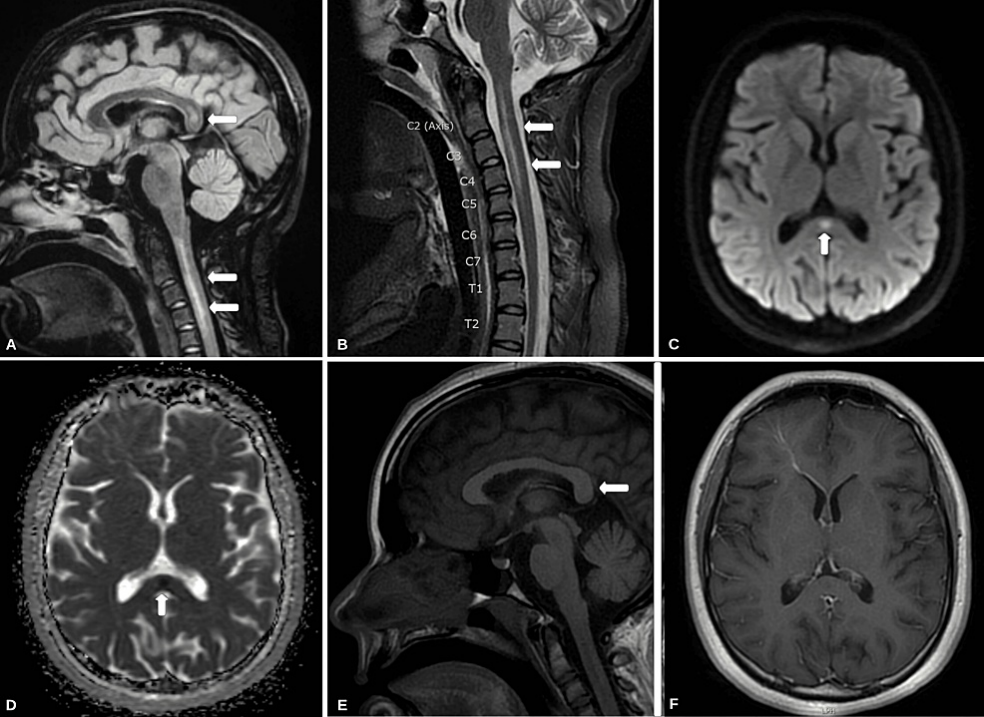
MRI of the brain and cervical spinal cord (A) T2 FLAIR sagittal sequence shows a non-enhancing lesion in the splenium of the corpus callosum (arrows). (B) T2 FLAIR sagittal sequence of the cervical spinal cord with hyperintensities from C1 to C5 (arrows). (C) DWI sequence depicts diffusion restriction within the splenium of the corpus callosum (arrow). (D) ADC sequence confirming the aforementioned diffusion restriction (arrow). (E and F) T1-weighted imaging with only mild hypointensity in the splenium of the corpus callosum (arrow) and without enhancement. MRI: magnetic resonance imaging, FLAIR: fluid-attenuated inversion recovery, DWI: diffusion-weighted imaging, ADC: apparent diffusion coefficient
